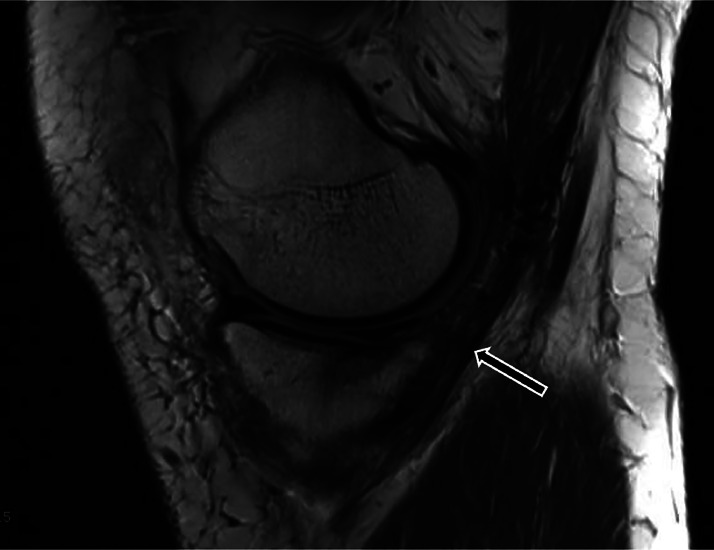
T2-weighted sagittal image 5 months post-repair. The arrow demonstrates the healed semimembranosus tendon.
